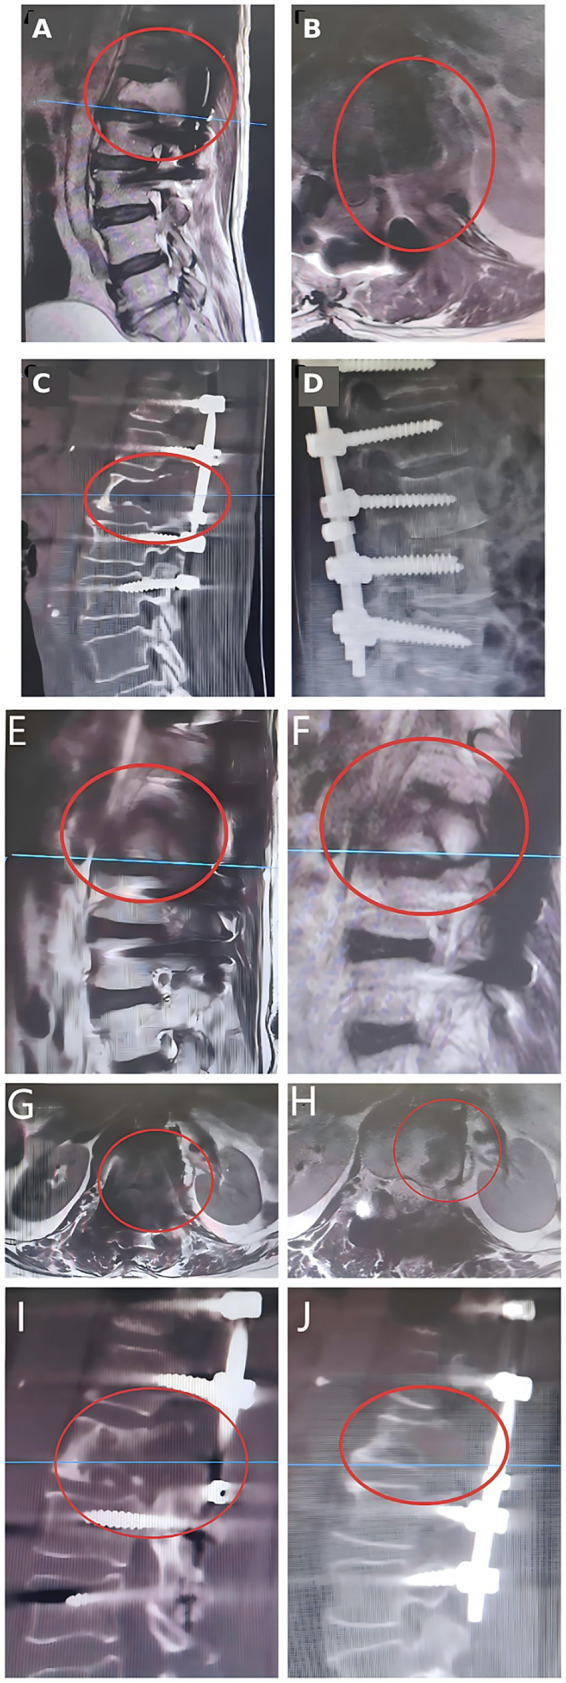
Early postoperative imaging (postoperative day 10) and follow-up imaging at 3 months and 1 year. (A) Sagittal lumbar MRI demonstrates expected postoperative changes after resection of the L1 lesion with T11–L3 posterior instrumentation; postoperative fluid/edema is present within the surgical bed (circle). (B) Corresponding axial MRI at the L1 level shows a mass-like postoperative signal abnormality within the left paravertebral/foraminal operative region (circle), compatible with early postoperative cavity/fluid and reactive change; residual lesion cannot be excluded on the non-contrast protocol. (C) Sagittal CT confirms postoperative osseous changes at L1 and the position of the posterior fixation construct. (D) Lateral radiograph shows satisfactory alignment and implant position without evidence of hardware failure. (E,F) Sagittal MRI at 3 months (E) and 1 year (F) demonstrates interval evolution of the surgical bed at the operated level (circle). (G,H) Corresponding axial MRI at 3 months (G) and 1 year (H) depicts the local postoperative anatomy at the same level (circle). (I,J) CT at 3 months (I) and 1 year (J) provides complementary assessment of osseous remodeling and postoperative stability (circle).
